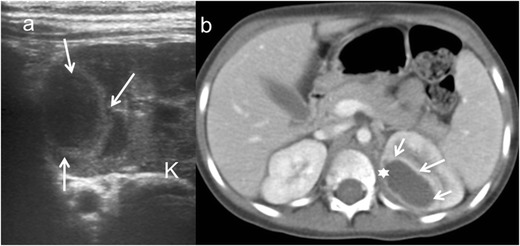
Renal abscess. A 5-year-old girl with fever. a Longitudinal sonographic image shows well-defined (*white arrows*) mass in upper pole of left kidney (*K*). b Contrast-enhanced nephrogenic phase transverse CT image shows hypodense lesion (*asterisk*) with rim enhancement (*white arrows*)
